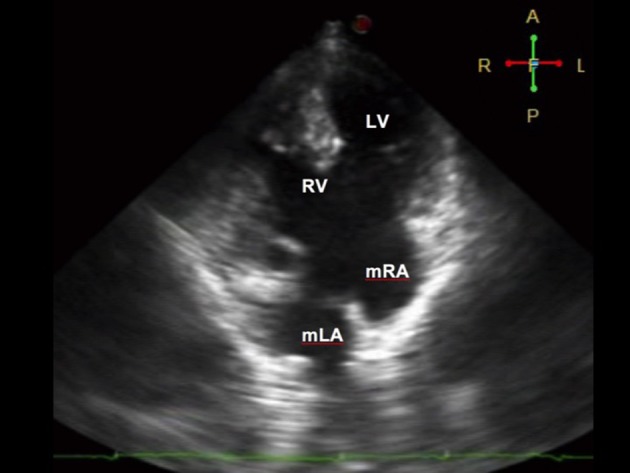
Four-chamber view on 2D echocardiography during diastole showing a complete atrioventricular (AV) septal defect Rastelli type A. There is a common AV valve, which makes it difficult to use the valves to identify the ventricles. Note that the moderator band is seen on the ventricle of the right (morphologic RV). The morphologic right atrium (mRA) drains into the left ventricle, while the morphologic left atrium (mLA) drains into the right ventricle, suggestive of atrioventricular discordance. The RV (systemic chamber) is more hypertrophied than the LV.
